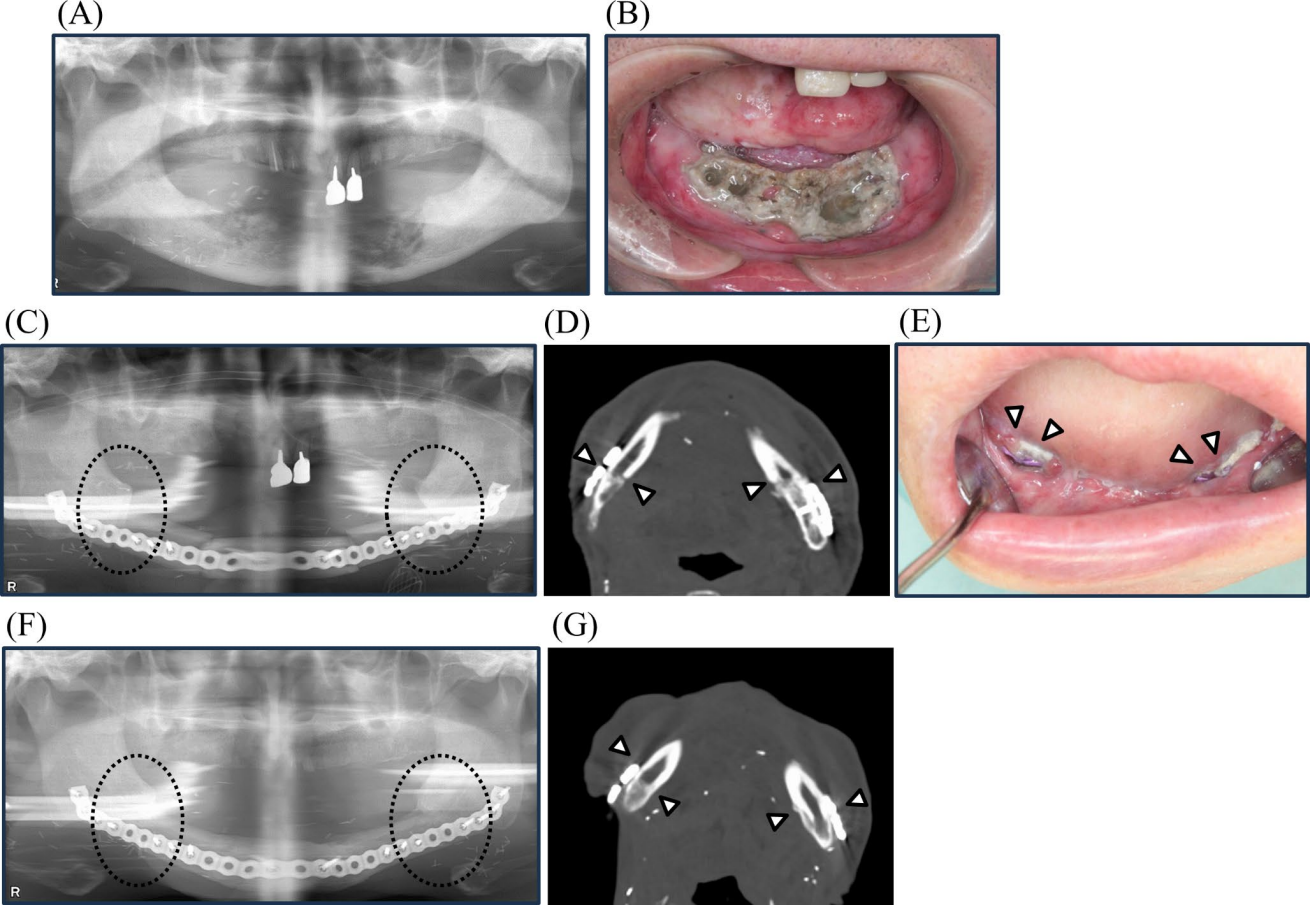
Radiological images and oral photo of a patient (No.15). (A)Panoramic radiograph image with advanced osteolysis before surgery. (B) Intraoral photo with bone exposure before surgery. The arrows show the part of bone exposure. (C) (D) Panoramic radiograph image at 2 weeks after surgery and CT image at 10 months after surgery. The circles and arrows show the bone joints of fibula flap and residual mandibles, which do not achieve bone union yet. (E) Intraoral photos with plate exposure at 8 days after surgery. The arrows show the part of plate exposure. (F) (G) Panoramic radiograph image and CT image at 4 years after surgery. The circles and arrows show the part of bone union
